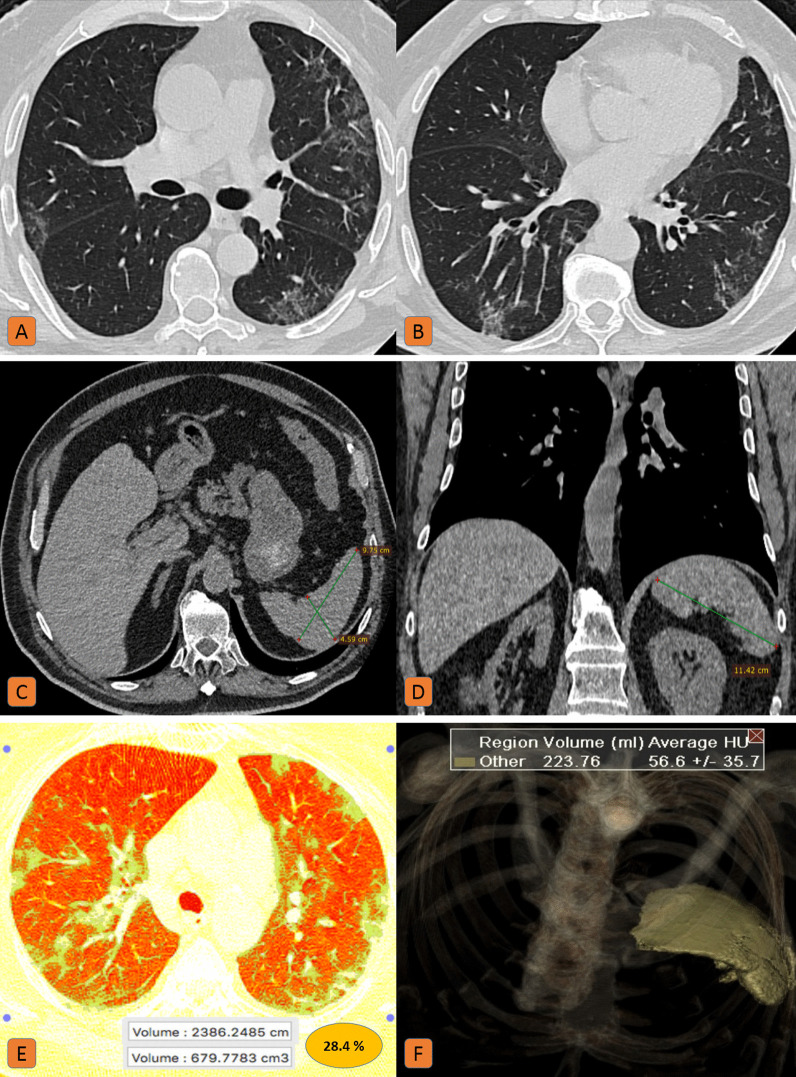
Normal sized spleen in a 74-year-old male patient with severe COVID-19 infection: A, B Axial chest CT (lung window) showed bilateral sub-pleural ground-glass patches with mild reticular thickening. C Axial, and D coronal CT cuts of the upper abdomen (soft tissue window) showed normal splenic diameters. E Lung volumetric CT assessment revealed 28.5% pathological lung involvement. F CT volumetry of the spleen was 223 cc
